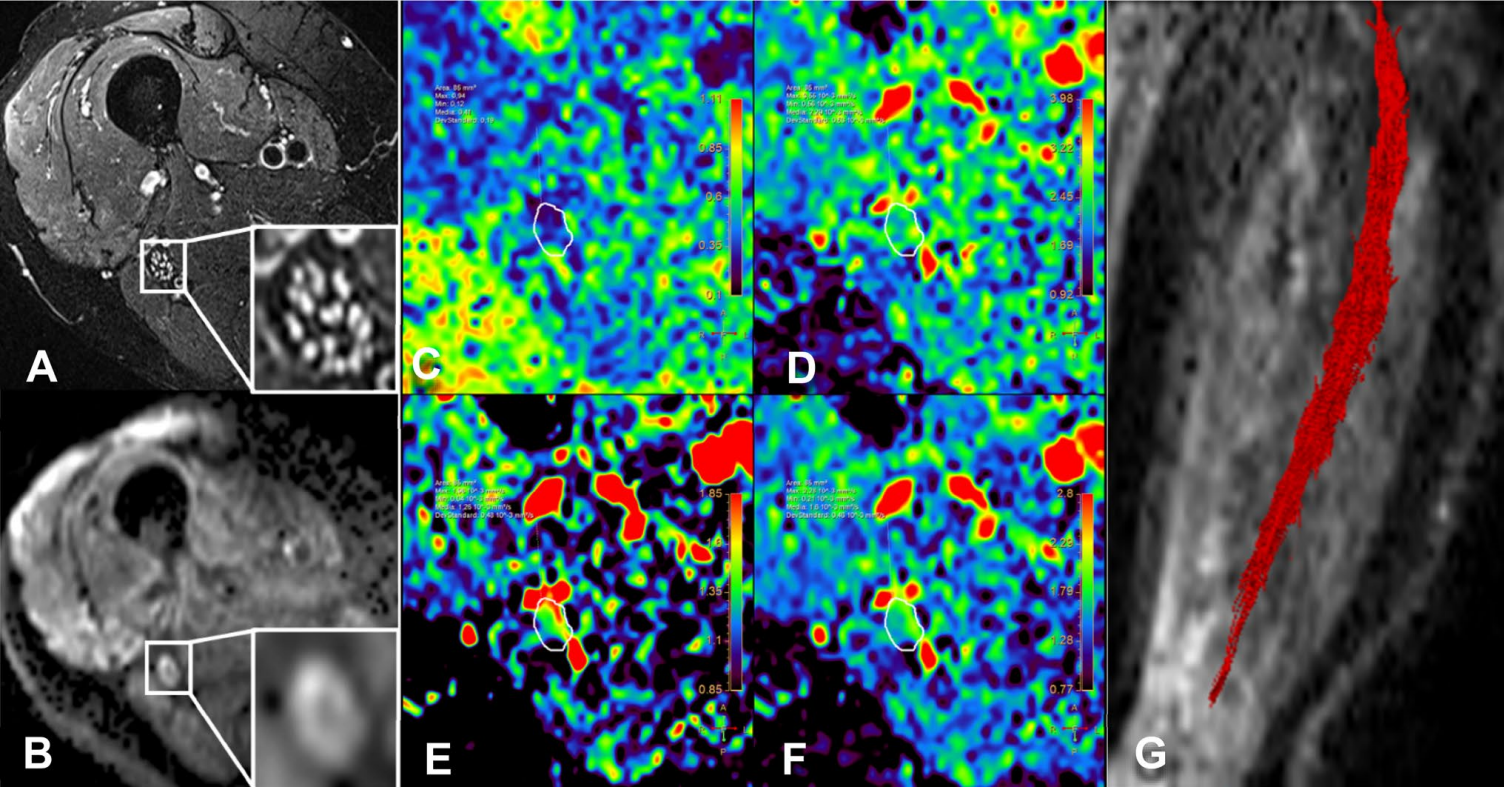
ATTRv-PN. MR Neurography, sciatic nerve at mid-thigh. B DWI trace-weighted image (*b* = 700), C FA, D AD, E RD, F MD maps, G sciatic nerve tractography at thigh. Sciatic nerve CSA 82.7 mm^2^, NSI = 2, FA = 0.41, AD = 2.29 10^–3^ mm^2^/s, RD = 1.26 10^–3^ mm^2^/s, ADC = 1.6 10^–3^ mm^2^/s. Increased signal intensity and size of the sciatic nerve, with significant reduction of FA
